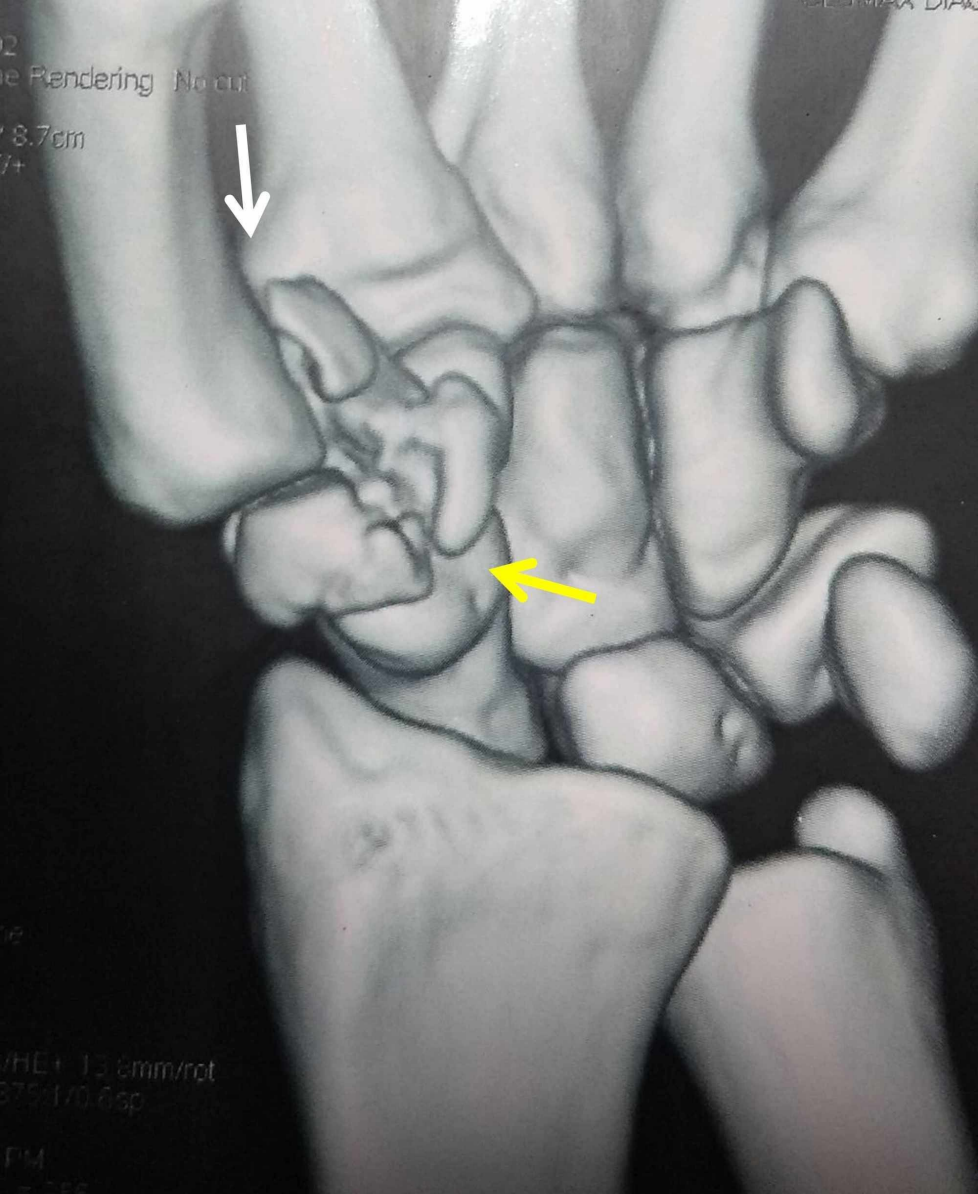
Three-dimensional computed tomography of the wrist White arrow - Bennett fracture; yellow arrow - trapezium fracture
